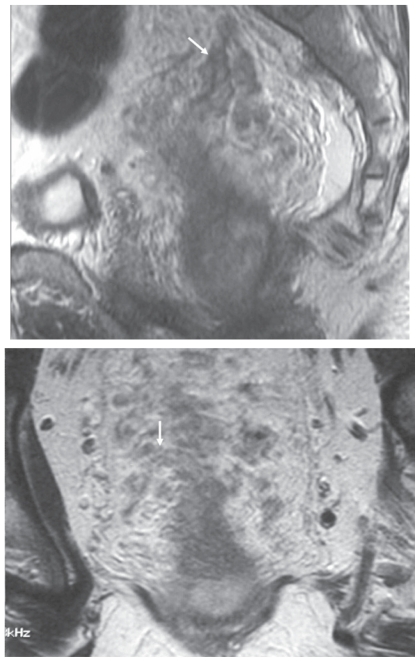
MR imaging demonstration of extramural venous invasion. T2-weighted imaging in the sagittal and coronal planes demonstrating multiple expanded serpingenous structures (arrows) of intermediate signal intensity emanating from the tumour. This is an example of grade 4 extramural vascular invasion.
